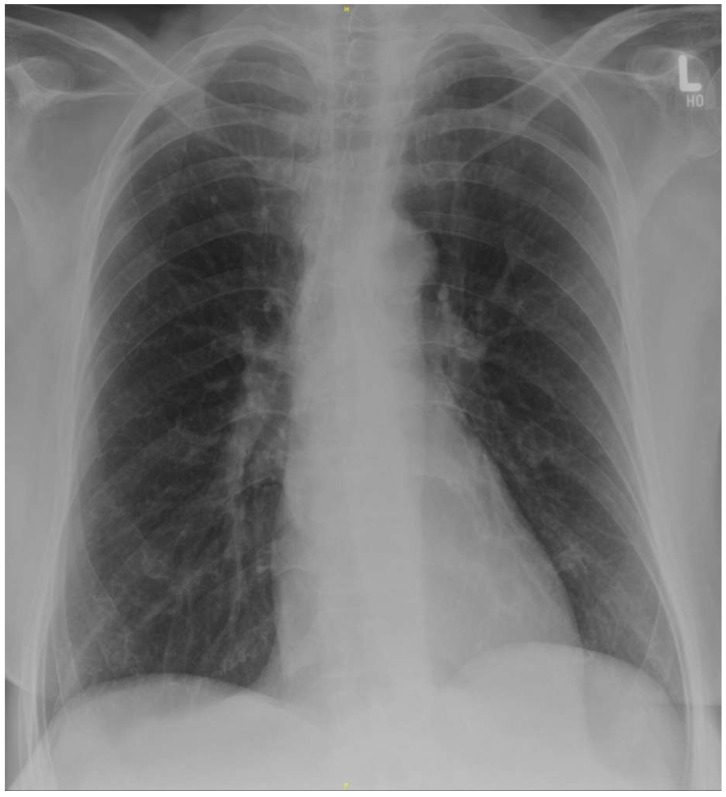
Supine chest X-ray at the time of admission without evidence of pneumonic consolidations or ground glass opacities. Courtesy of Prof. Gerhard Adam, Department of Diagnostic and Interventional Radiology and Nuclear Medicine, University Medical Center Hamburg-Eppendorf, Hamburg.
